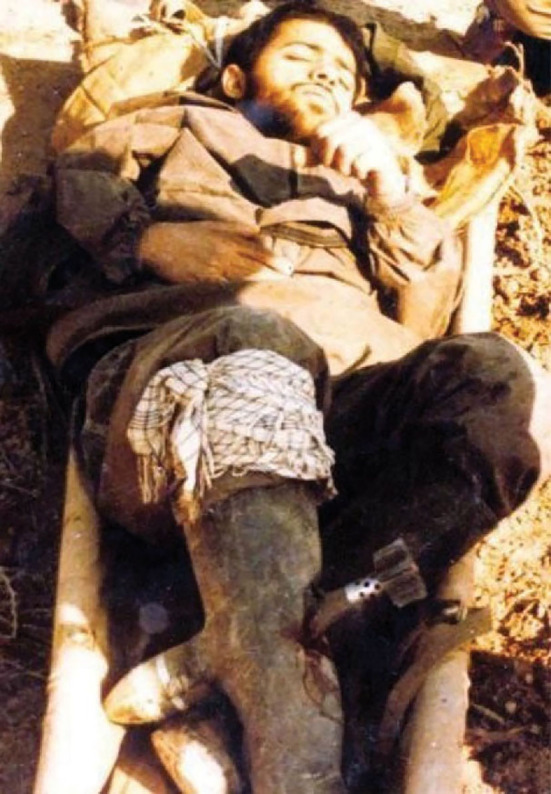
The above-mentioned report incited us to present another case of impaled unexploded mortar shell during the Iran-Iraq war. On August 24, 1985, the left upper limb of an Iranian combatant was hit with a 60mm mortar shell while he was talking on a communicating device. The unexploded mortar sewed the left forearm and arm together (Figure 2). Doctor Mohajer removed the impaled unexploded mortar shell in the operating room under general anesthesia. Plain radiographs demonstrated that the humerus, radius and ulnar bones were fractured; however, there was no neurovascular injury. The patient was further treated by Dr. Shojaedin Sheikholeslamzadeh.^1^
